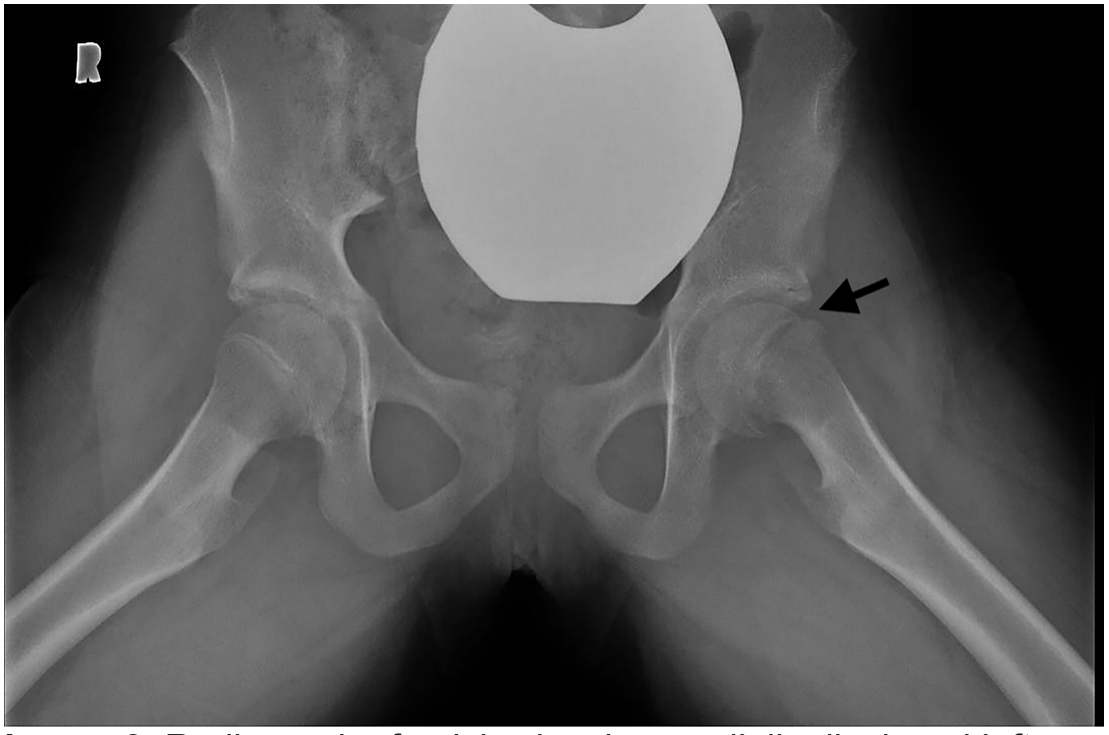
Radiograph of pelvis showing medially displaced left femoral epiphysis (arrow).
